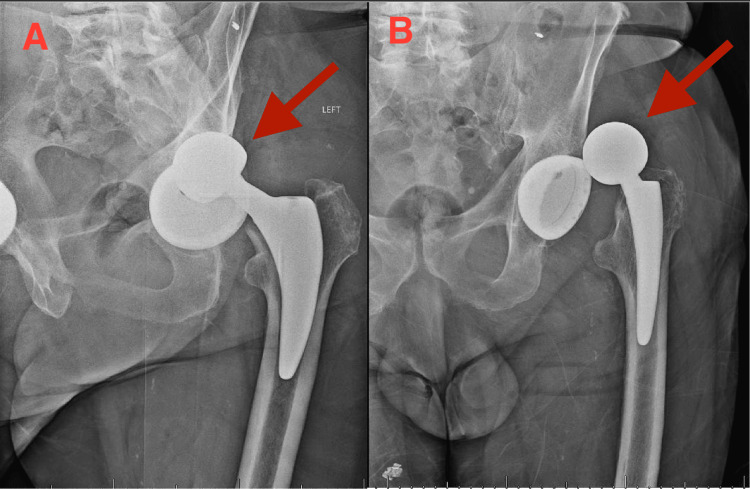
AP left hip radiograph (A) and oblique left hip radiograph (B) demonstrating anterior dislocation of left THA AP, anterior-posterior; THA, total hip arthroplasty
